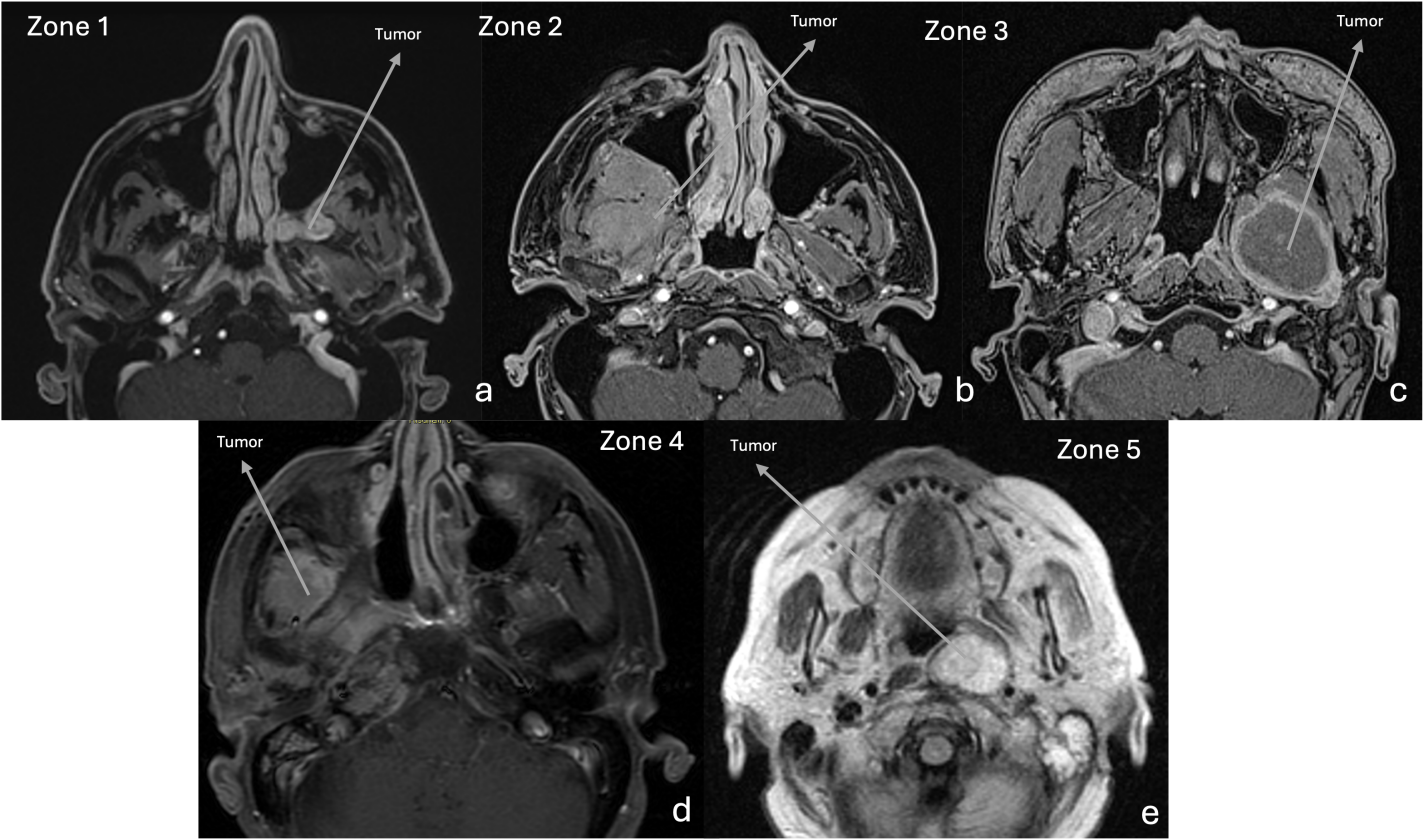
MRI. Axial T1-w after gadolinium, showing the tumor location, according to Li et al. ^26^
